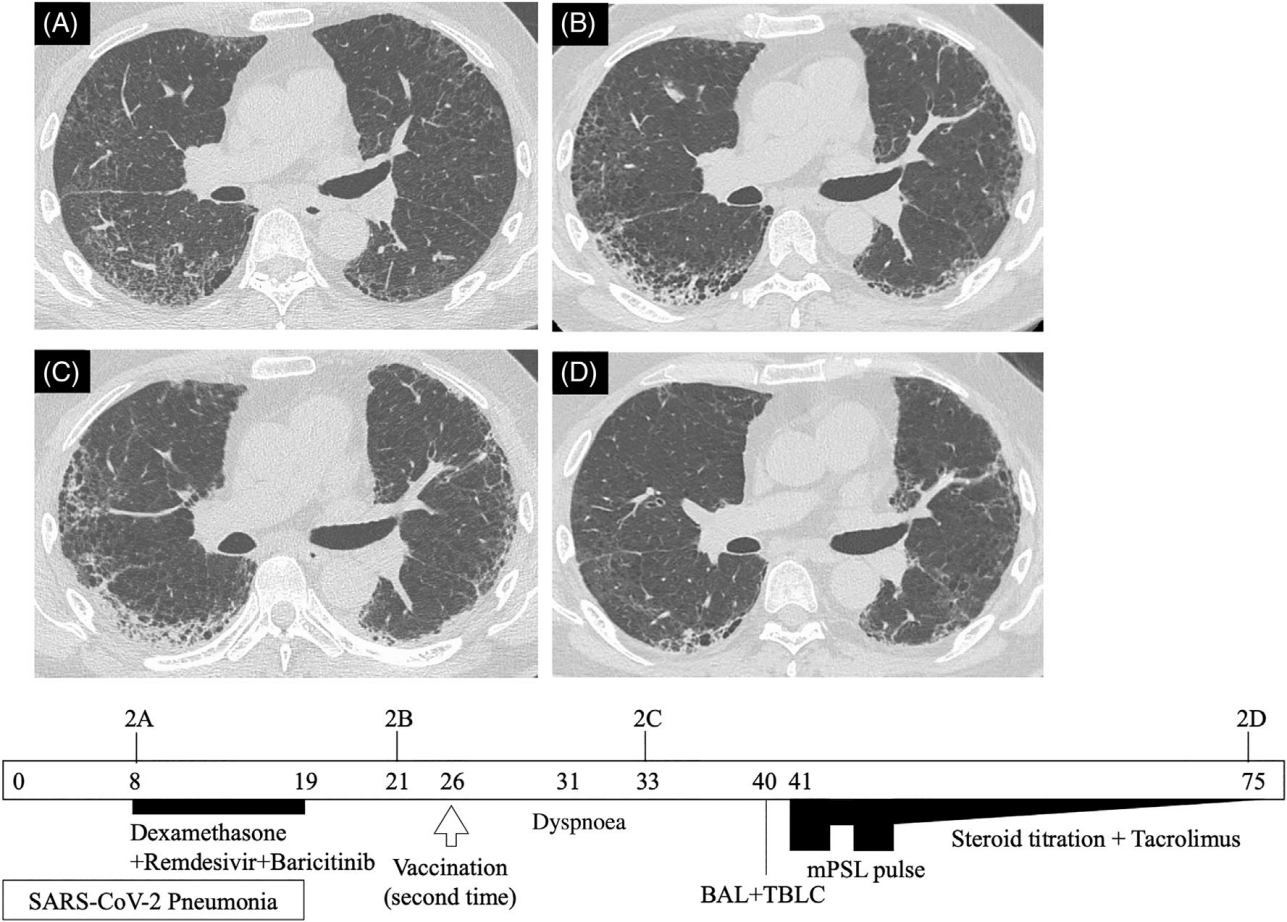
Simple clinical course of Case 2. Each HRCT corresponds to the figure numbers in the main text. Arabic numerals in the timeline square indicate the number of days since the onset of COVID‐19. BAL, bronchoalveolar lavage; COVID‐19, coronavirus disease 2019; HRCT, high‐resolution computed tomography; mPSL, methylprednisolone; SARS‐CoV‐2, severe acute respiratory syndrome coronavirus 2; TBLC, transbronchial lung cryobiopsy
